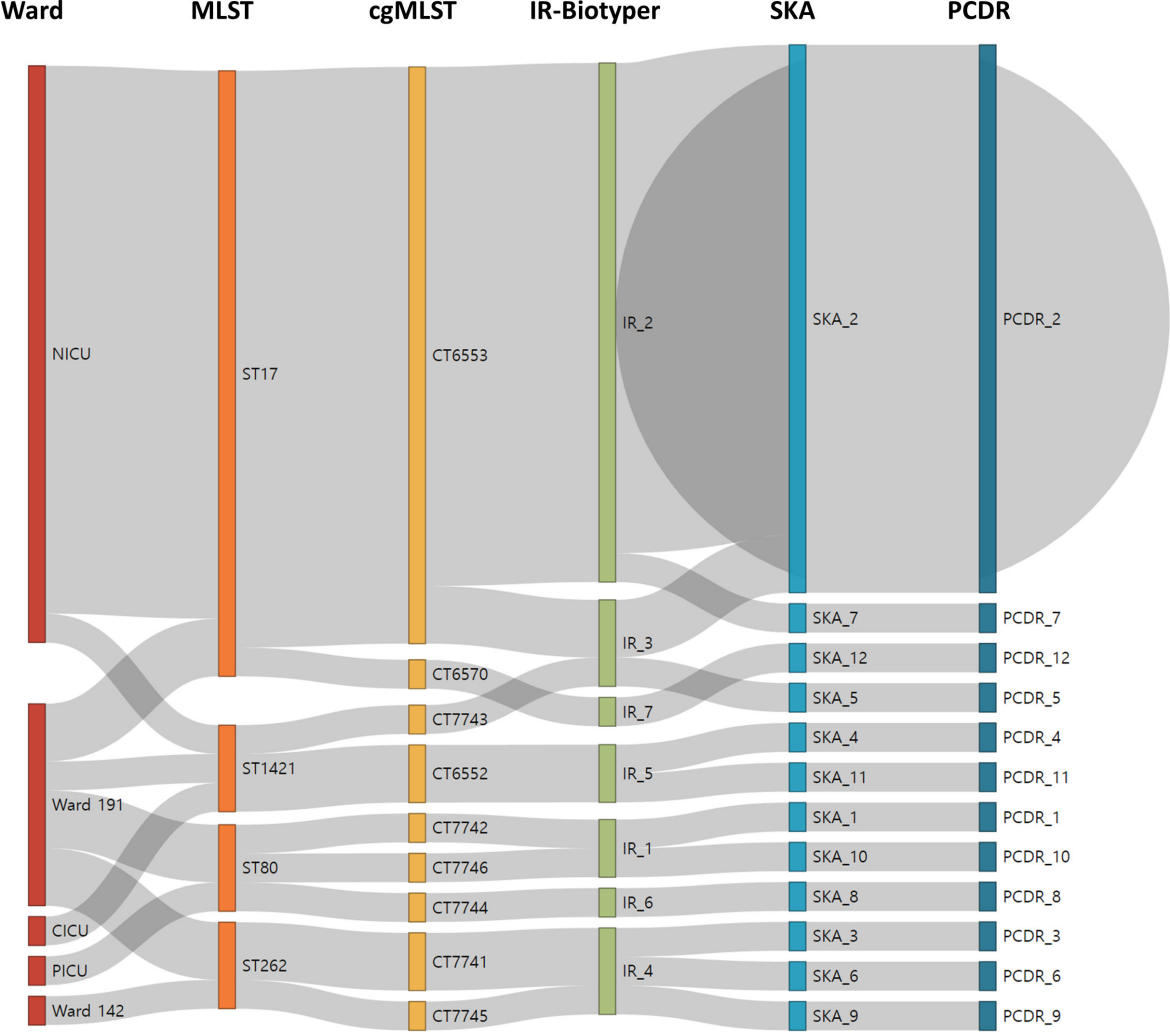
Relationship between methods for clustering (*n* = 30). Abbreviations: PICU, pediatric intensive care unit; NICU, neonatal intensive care unit; CICU, cardiac intensive care unit; EFM, *Enterococcus faecium*; ST, sequence type; CT, complex type.
